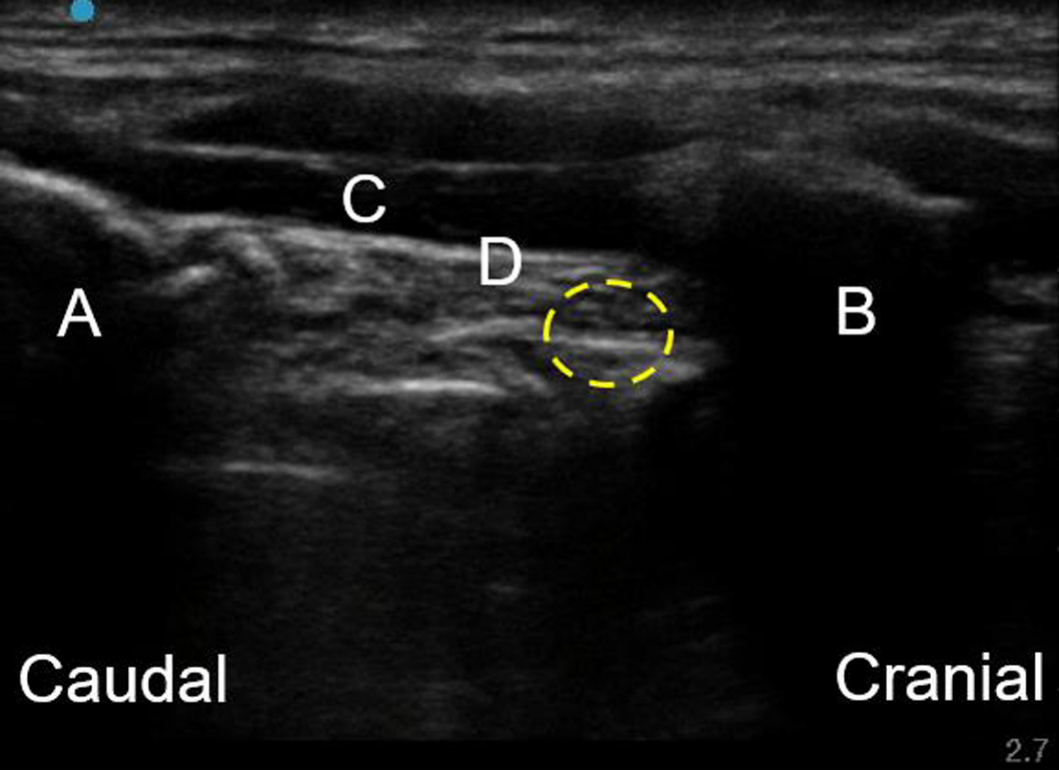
Ultrasound images of structures around the superior laryngeal nerve. Thyroid cartilage (A) hyoid bone, (B) thyrohyoid muscle, (C) and thyrohyoid membrane, (D) are marked on the ultrasound image. The Yellow dotted circle represents the space of the superior laryngeal nerve.
